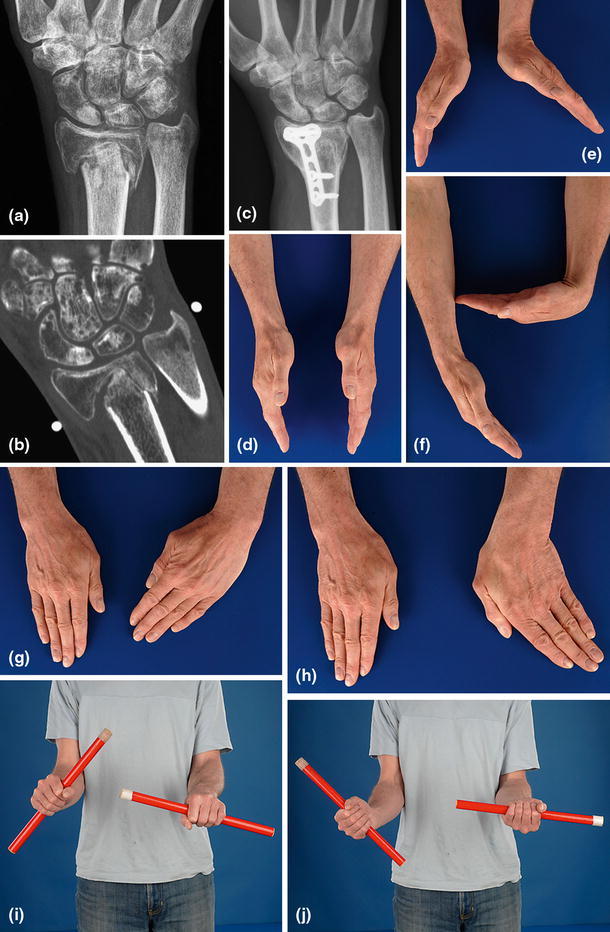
a, b Plain AP radiograph and CT of a distal radius nonunion. Detail proved by CT facilitates pre-operative planning; c Placement of a tricortical graft allow for some correction of radial length. Intrinsic stability provided by the soft tissue tensioning increased stability in the nonlocking era; d–j Wrist and forearm function at 7 years follow up
